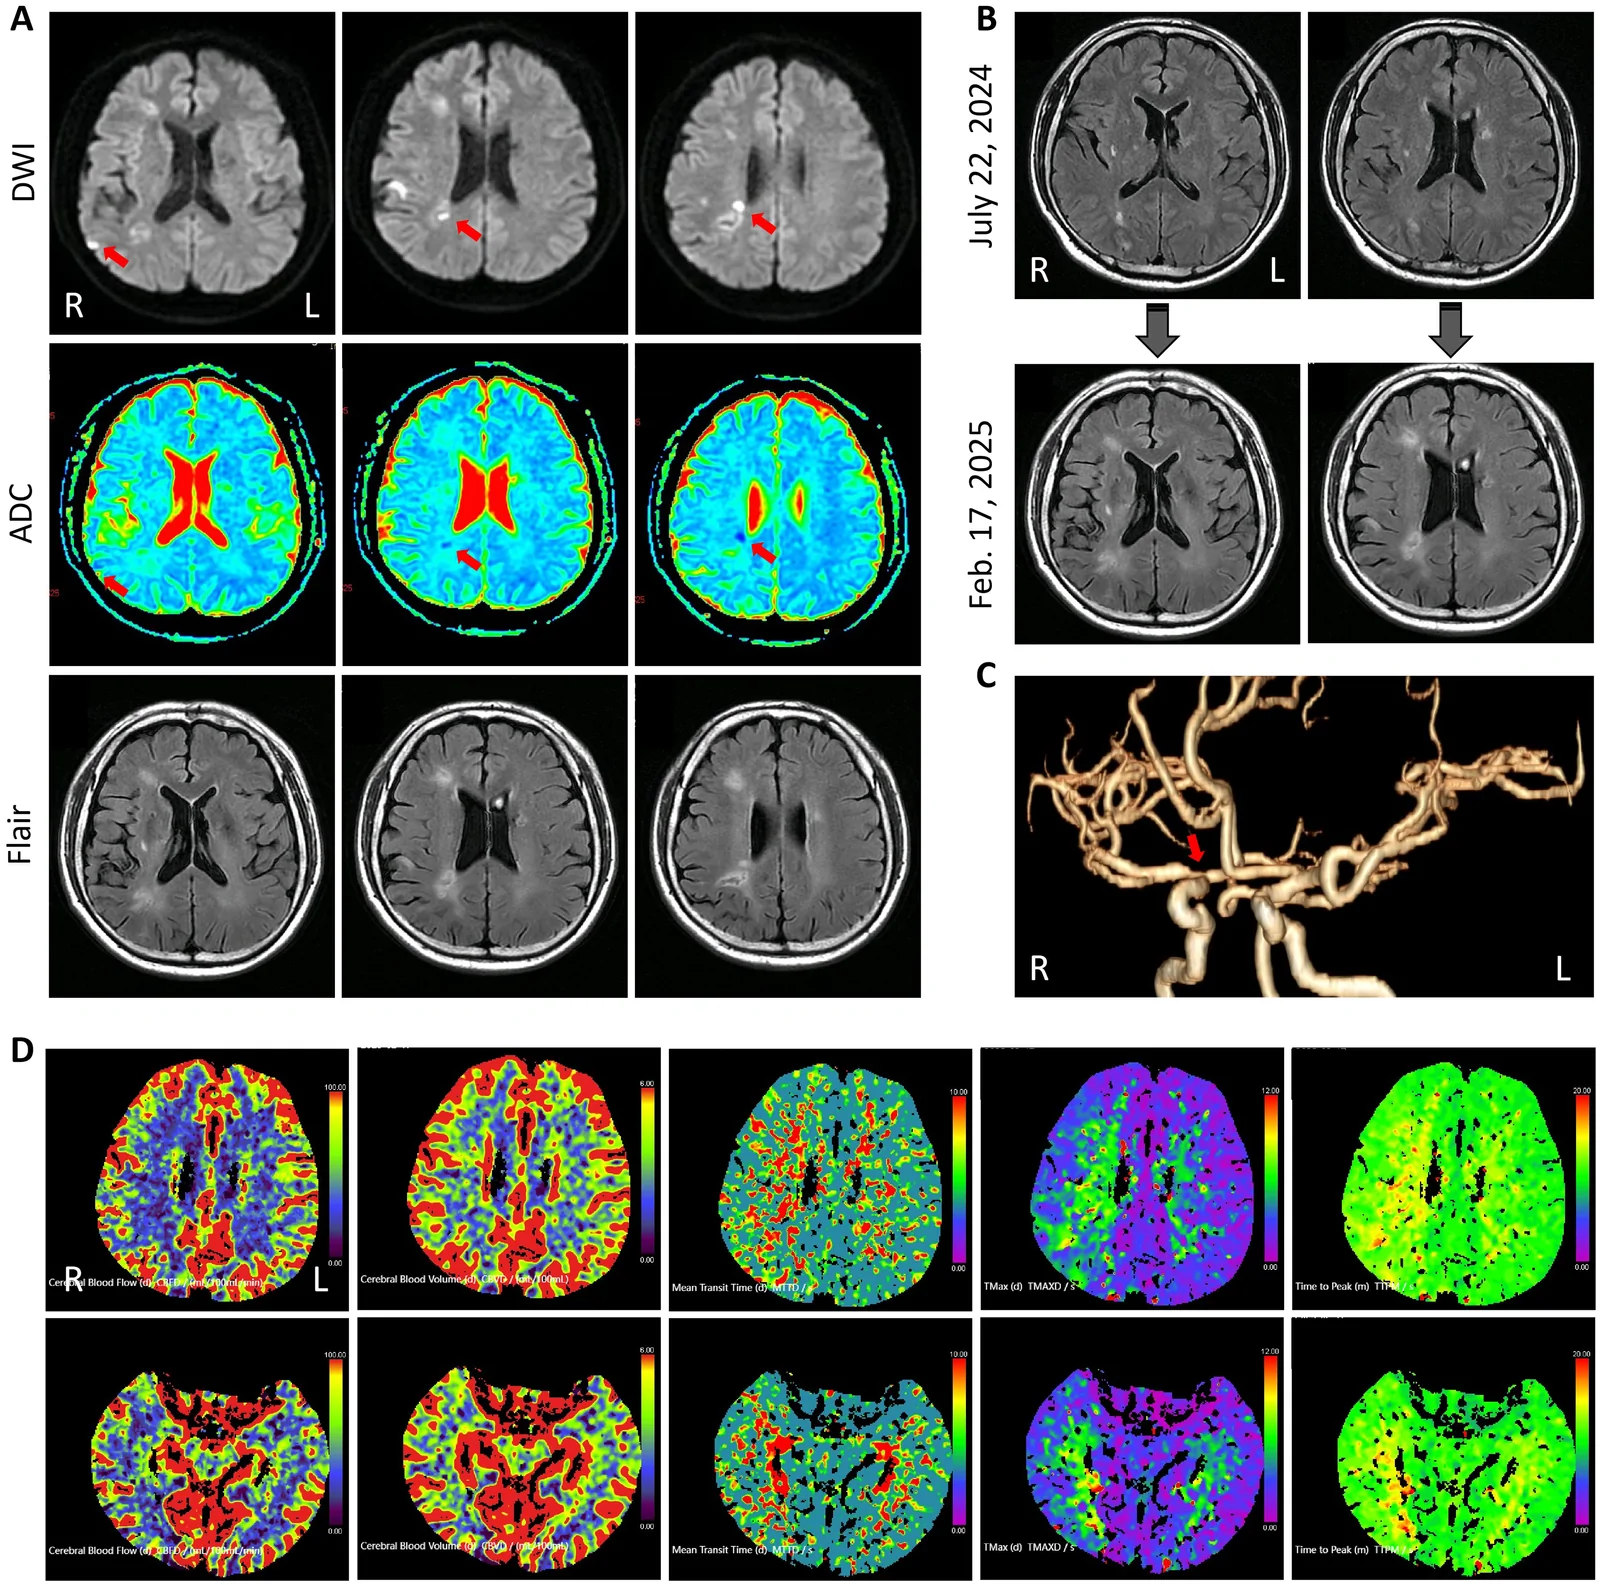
Neuroimaging findings from the second admission (Case 1). The patient was readmitted seven months after the initial stroke with recurrent left-sided weakness. (A) Brain MRI reveals multiple patchy acute infarcts involving the deep white matter of the right frontal and occipital lobes (red arrows). The panels are arranged from top to bottom as DWI, ADC, and FLAIR, respectively. (B) Comparison of FLAIR sequences between the two admissions demonstrates a marked interval increase in white matter hyperintensities, indicating progression of chronic microvascular pathology. (C) The CTA shows severe luminal stenosis of the right MCA at the M1 segment (red arrow), representing significant atherosclerotic progression compared to the prior study. (D) The CTP maps reveal prolonged MTT and TTP in the cortex and subcortical white matter of the right frontal, parietal, occipital, and temporal lobes, corresponding to the MCA territory, while CBV and CBF remain relatively symmetric to the contralateral side. These findings are consistent with hemodynamic compromise and perfusion insufficiency without overt irreversible ischemic core expansion. FLAIR, fluid-attenuated inversion recovery; CTA, computed tomography angiography; MCA, middle cerebral artery; CTP, CT perfusion; MTT, mean transit time and TTP, time-to-peak; CBV, cerebral blood volume; CBF, cerebral blood flow.
